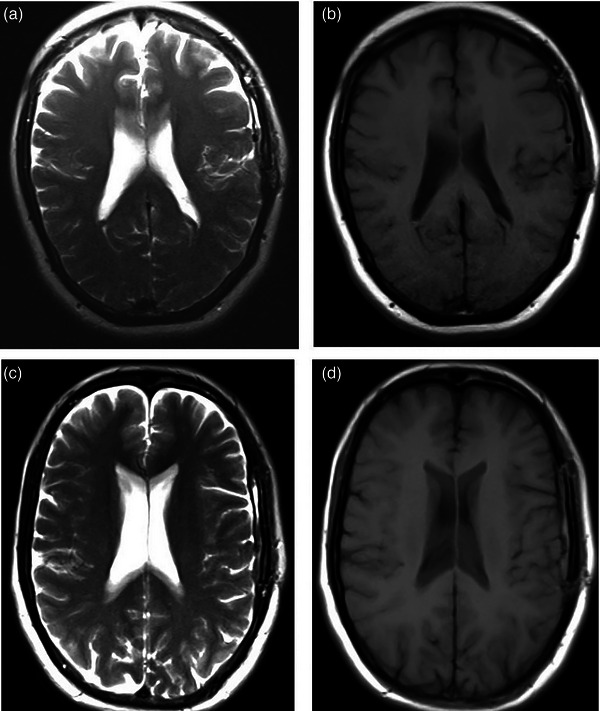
(a) T2 weighted and (b) T1 weighted MRI in RT setup; (c) T2 weighted, and (d) T1 weighted MRI in DS setup.
